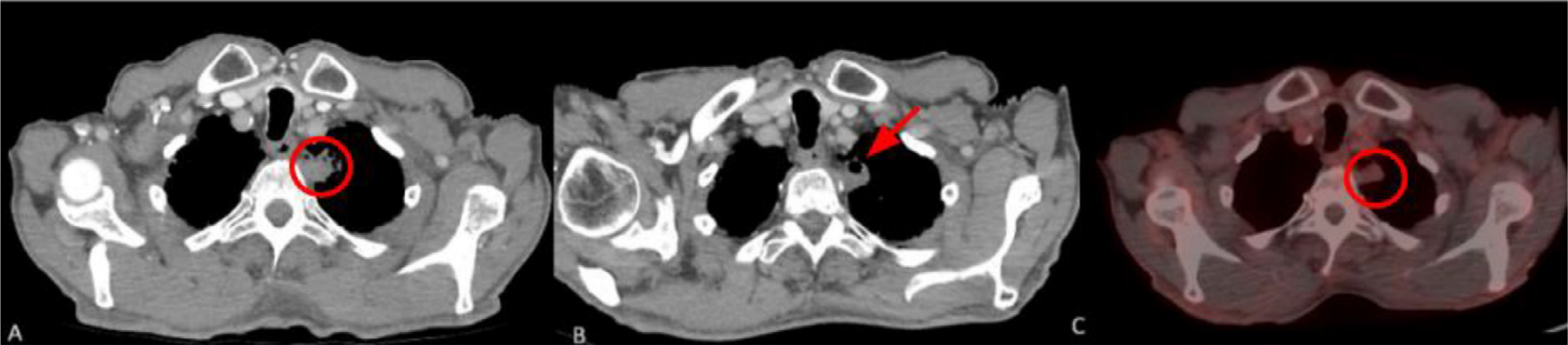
Follow-up images from the second patient. (A) 1-month postablation thoracic CT scan showing no enhancement of the ablated lesion consistent with absence of residual tumor or local recurrence. The area demonstrates a nodular pattern (area into the circle). (B) The thoracic CT 3 months after the cryoablation shows a small cavitation in the ablated nodule (red arrow), which is common as it appears in about half of the patients and is typically resolved within 6 months. (C) The PET/CT scan 6 months postcryoablation revealed no Fluorodeoxyglucose (FDG) uptake of the lesion (area into the circle), as well as no evidence of distant metastases .
